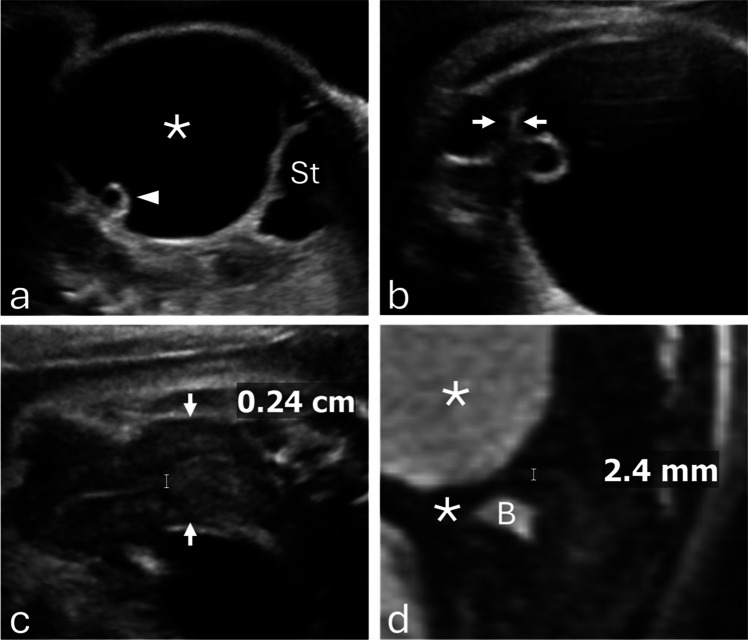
a Prenatal sagittal grayscale ultrasound at 36 weeks 5 days demonstrates a large unilocular ovarian cyst (asterisk) extending from the left pelvis to the upper abdomen adjacent to the stomach, containing a “daughter cyst” (arrowhead), characteristic of ovarian cyst. b A close-up image demonstrates peripheral displacement of the ovarian stroma (arrows). c Sagittal grayscale ultrasound shows the fetal uterus (arrows) with a thickened endometrium measuring > 2 mm. d Sagittal T2-weighted MR image confirms a thickened endometrium. The infant underwent exploratory laparotomy with cyst drainage, which confirmed a simple ovarian cyst
